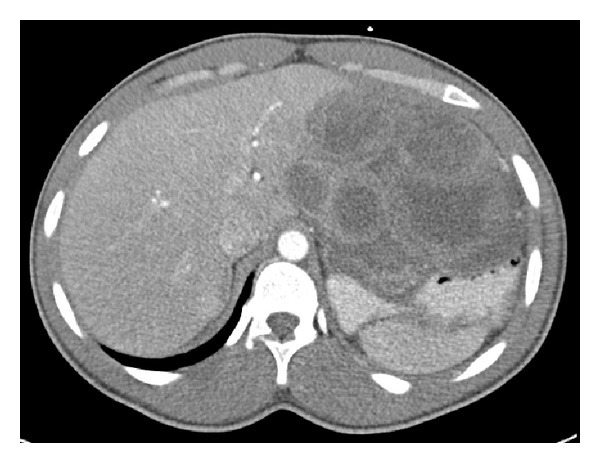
CT image showing increase in tumor size four months after doubling the dosage of imatinib to 800 mg per day.
